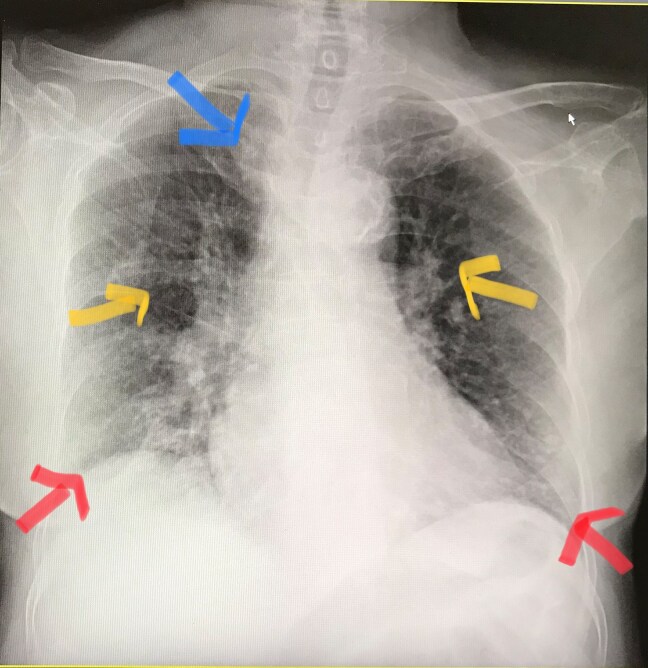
Chest radiograph demonstrating bilateral pleural effusions, interstitial lung involvement, and consolidation in the right upper lobe.
